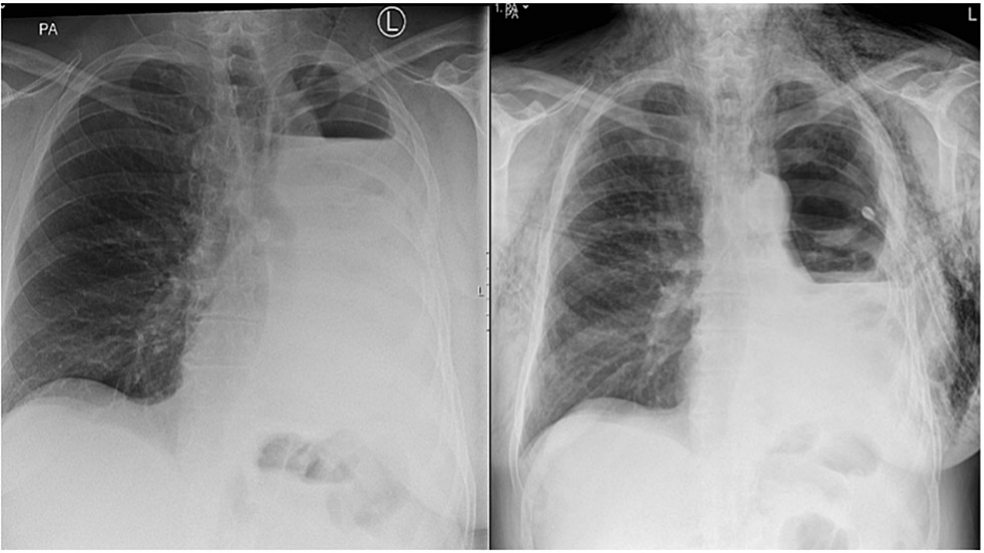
A comparison of the chest radiograph after pneumonectomy prior to hospital discharge (left) with that following clinic review five weeks later (right)
